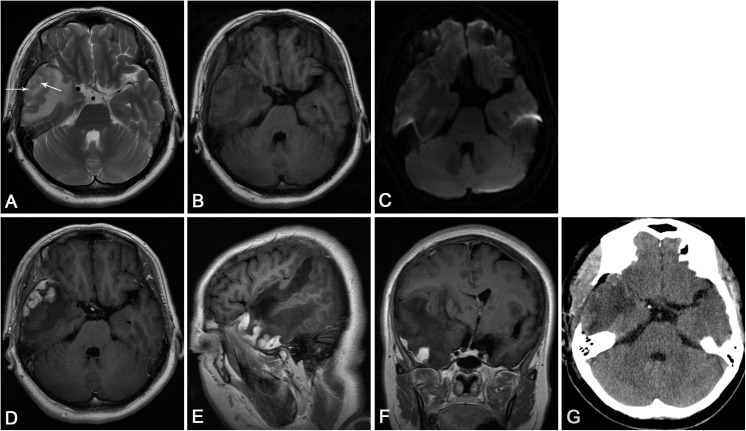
(A) Axial T2-weighted imaging showed multiple heterogeneous hypersignal nodules with central spots of hyposignal (arrows) in the right temporal lobe and significant edema around the lesion. (B) Axial T1-weighted imaging revealed the heterogeneous hyposignal nodules. (C) Diffusion-weighted imaging revealed slight hyperintense nodules. (D) Axial and (E) sagittal T1-weighted images with contrast demonstrated the enhancing nodules arising from the meninges, with significant perilesional edema. (F) Coronal T1-weighted images with contrast showed the mass effect of the nodules, which led to the compression of the right lateral ventricle and evident midline shift. (G) Post-operative CT showed that no nodules with a certain amount of hypodense edematous zone could be found on the right temporal lobe.
